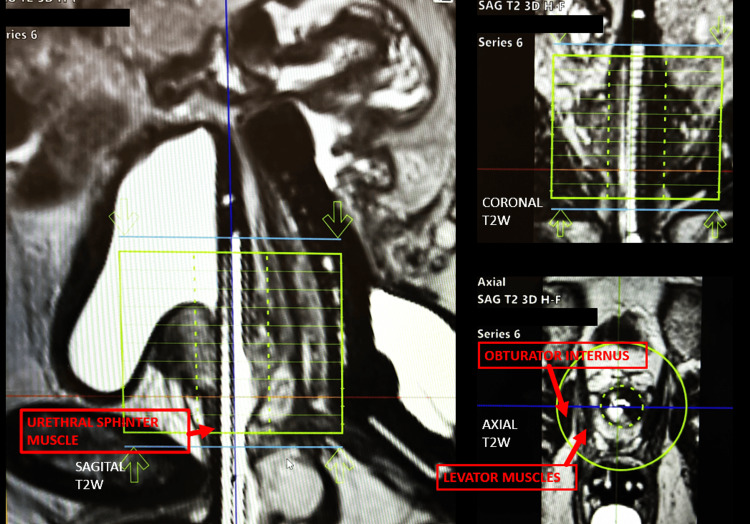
TULSA-PRO software and prostatic anatomic landmarks Representative axial, coronal, and sagittal planning images from the transurethral ultrasound ablation of the prostate (TULSA-PRO) platform demonstrating the urethral applicator, rectal cooling device, and key anatomic landmarks relevant to treatment of apical prostate tumors, including the levator ani and obturator internus muscles. Accurate identification of these structures is critical for treatment planning and preservation of continence.
